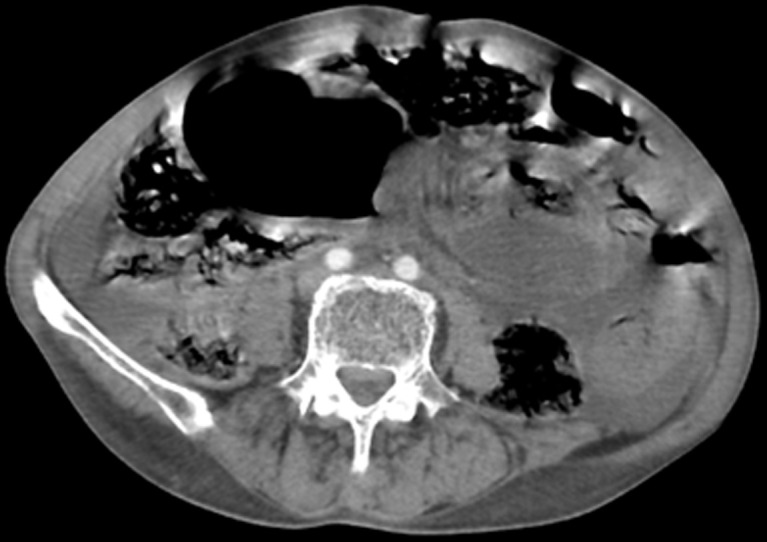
a computed tomography scan showing a radial arrangement of the small bowel in the left lower quadrant with a transition zone, associated with a sign of whirlpool
